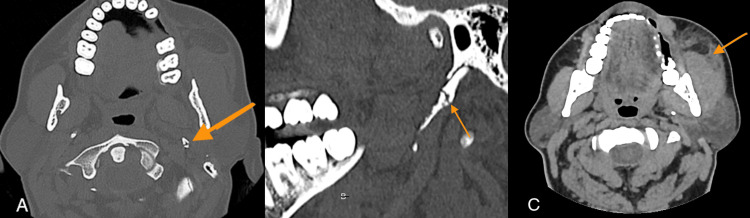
Maxillofacial CT images. Axial (A) and sagittal (B) images show a non-displaced fracture of the left styloid process (yellow arrows). Soft tissue swelling and hematoma in the left masseter muscle are also detected on soft tissue window CT image (C). CT: computed tomography.
